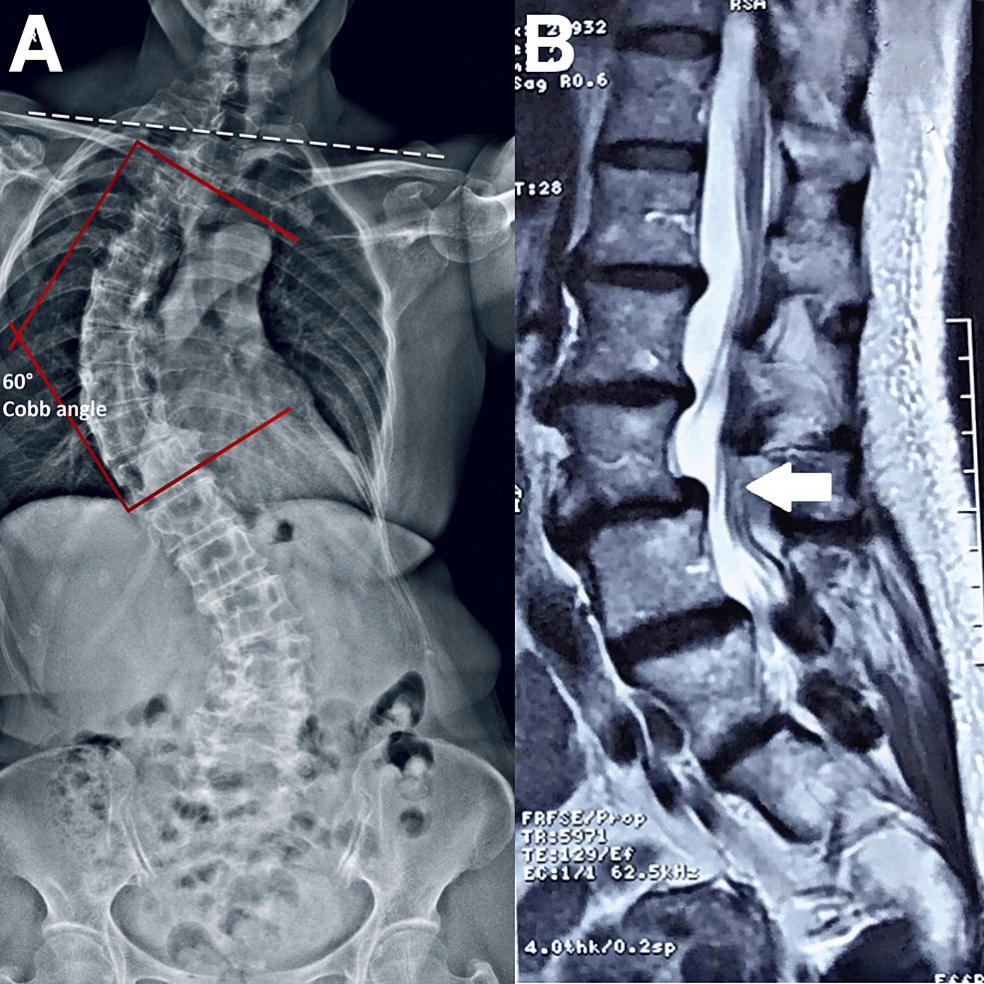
Full-spine radiograph and lumbar magnetic resonance image A) Full-spine radiograph revealing degenerative changes in the thoracic and lumbar regions, shoulder and pelvic imbalance (dash white line), and abnormal thoracic and lumbar scoliotic curves. The Cobb angle was measured at 60 ° (red lines) B) At L4-5 (white arrows), there are spondylolisthesis, posterior bulging disc, and marked bilateral facet joint degeneration, in the right facet joint and ligamentum flavum hypertrophy causing mild indentation of the thecal sac. Diffuse bulging disc and osteophytes at L2-3 causing mild indentation of the thecal sac, mild right lateral recess and mild bilateral foraminal stenosis. No nerve root compression is seen.
